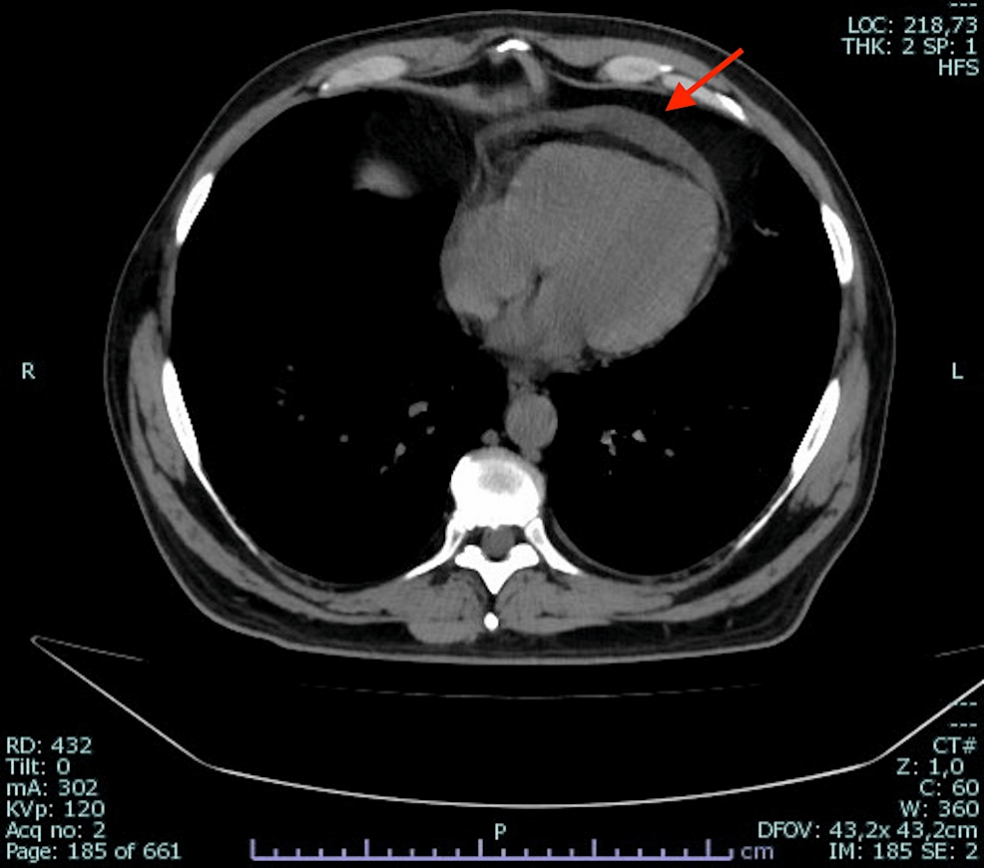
Chest CT shows a pericardial effusion with a diameter of 1.9 cm (arrow).
